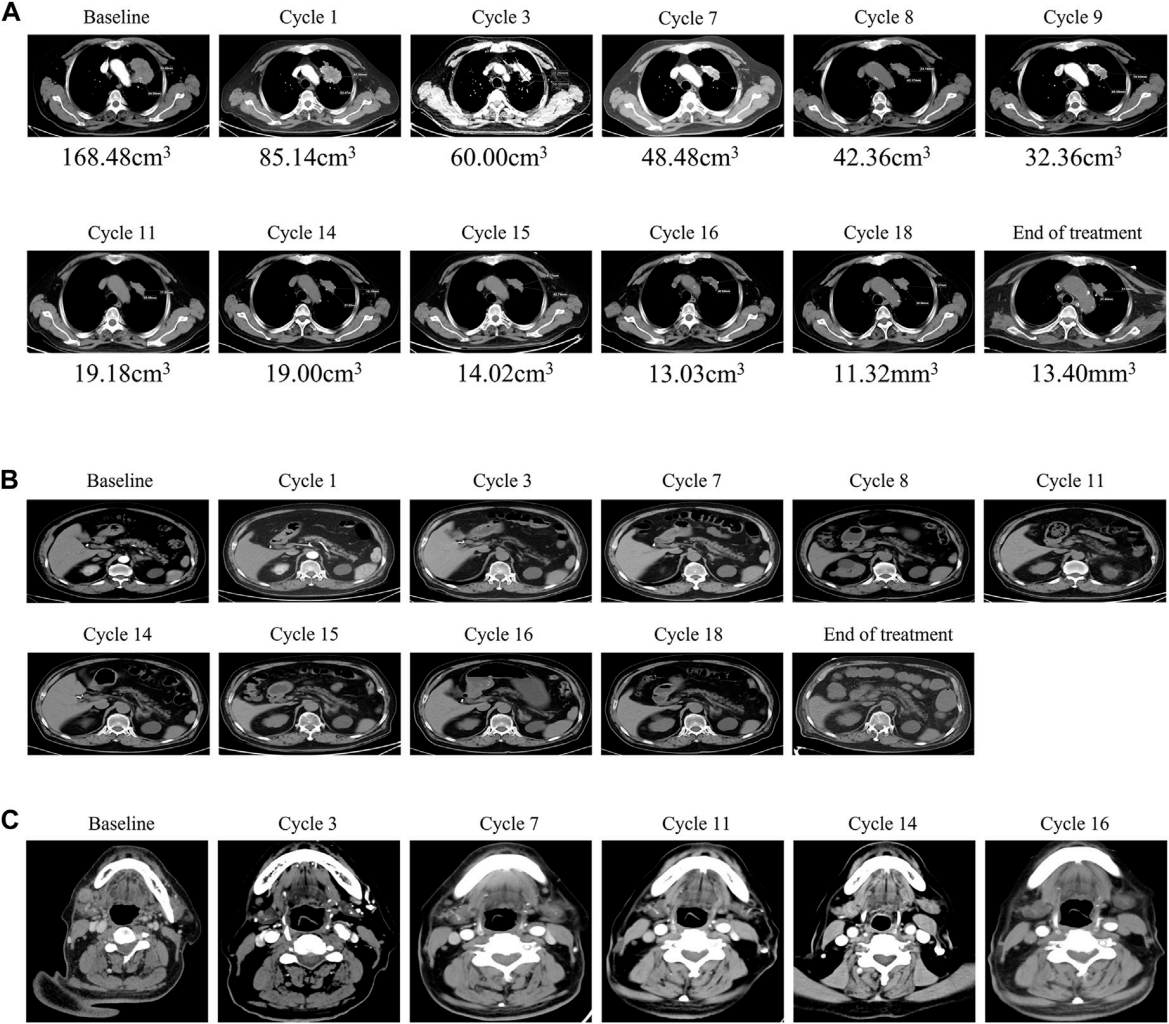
Changes in lung tumor and metastatic tumor during camrelizumab and anlotinib treatment. (A) CT showed that the lung tumor began to shrink after first cycle of treatment until the end of treatment. (B) CT showed that the volume of bilateral adrenal invasion lesions was significantly reduced after first cycle of treatment and disappeared after that. (C) CT showed that the volume of the right neck invasion lymph node lesion was significantly reduced to normal after three cycles until the end of treatment.
